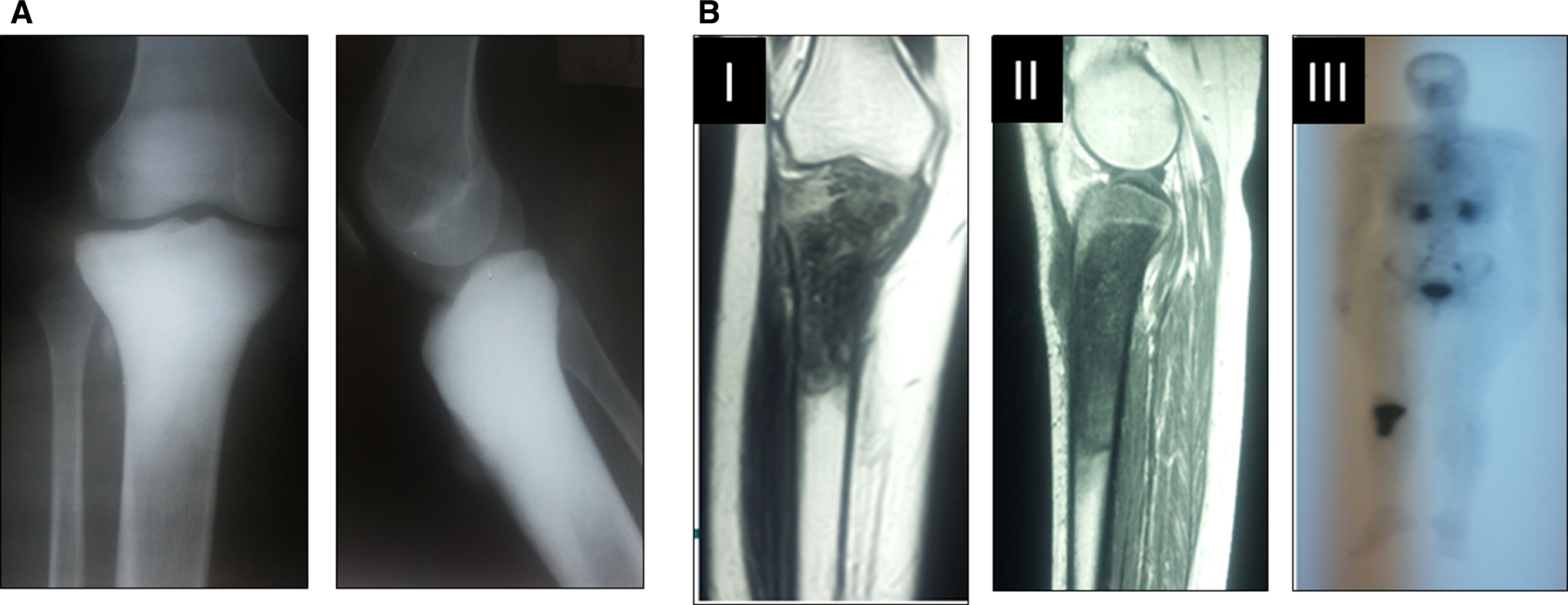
a Plain radiograph anteroposterior and lateral view of the knee showing osteoblastic lesion of the proximal tibia. b MRI cuts (I) coronal cut (II) sagittal cut showing medullary involvement and soft tissue extension and bone scan (III) showing hot spot
